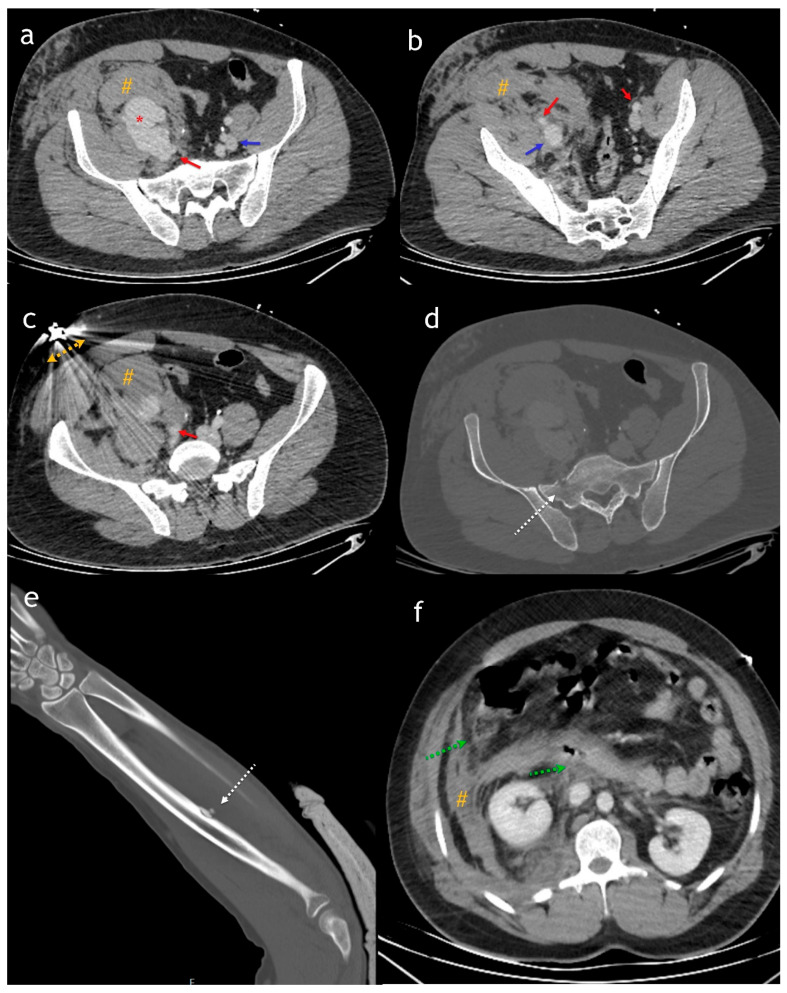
Axial (a–c) images from an abdominal CT scan obtained in the portal phase [soft tissue window] show the iliac artery [red arrow], iliac vein [blue arrow], a large hematoma [#], and a suspected pseudoaneurysm [*]. However, due to the acquisition phase, both iliac veins appear similar, making the AVF undetectable. In the bone window (d), a secondary fracture [dashed white arrow] is observed in the sacrum and left radius (e), caused by the bullet’s trajectory. In the upper abdominal sections (f), more pronounced fat stranding around the duodenum and increased wall thickness in the duodenum and cecum [dashed green arrow] and hematoma [#] raise suspicion of hollow viscus injury. Arterial phase CTA is the most effective diagnostic modality for AVF, as it demonstrates an enhancing venous lumen with the same density as the accompanying artery, significantly more than other veins [1,2]. Additionally, the direct connection of a pseudoaneurysm with both the injured artery and vein can be better visualized. The venous phase is also useful, as it may reveal solid organ injuries. If contrast media extravasation is present, indicating active hemorrhage, the volume of extraluminal contrast increases in the venous phase [1,2]. Gunshot wounds to the abdomen carry a high risk of vascular injury, and high-quality imaging is essential for accurate diagnosis. Biphasic computed tomography angiography (CTA), which includes arterial and portal venous phases, is the gold standard for evaluating both vascular structures and solid organ injuries [3,4]. The arterial phase is particularly crucial for detecting arterial injuries, pseudoaneurysms, and active contrast extravasation, while the portal venous phase enhances the visualization of solid organ injuries, venous abnormalities, and secondary findings such as bowel wall thickening or hematomas. Proper timing in imaging is crucial, as in this case, an initial miscalculation led to diagnostic difficulties.
